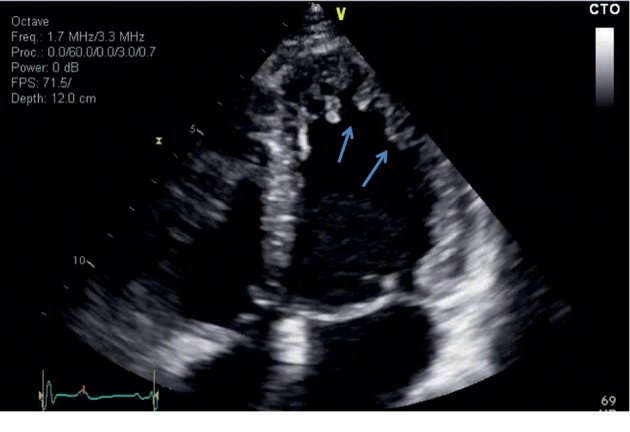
Three months after the second delivery: transthoracic echocardiographic apical 4-chamber view showed hypertrabeculation in the LV apex and lateral wall.
